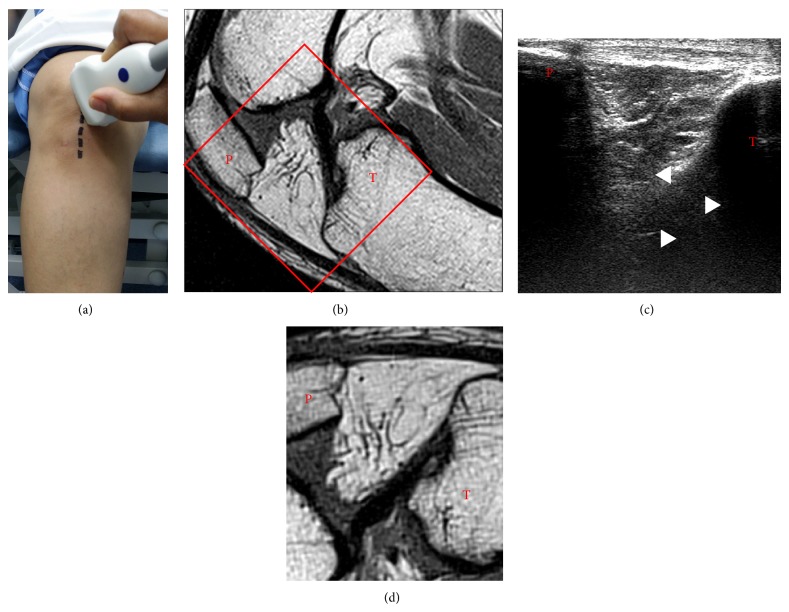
Position of the patient and the ultrasound probe during ultrasound examination of anterior cruciate ligament (ACL) is shown in (a). MRI image of knee in 90-degree flexion is shown in (b). The red rectangle denotes area of the image rotated and presented in (d) to match the morphology as seen in ultrasound image (c). White arrowheads in (c) demonstrate normal ACL. P denotes patella and T denotes tibia.
